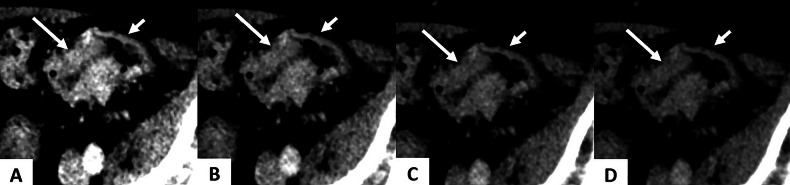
54-year-old male with pT3 sigmoid adenocarcinoma. DS-DECT (Siemens Force in axial section at VP with VMI at different energy levels. A 40 keV, B 50 keV, C 60 keV, D 70 keV. 40 keV VMI image shows improved contrast between high enhancement of the tumor (long arrow) and adjacent enhancement of normal bowel (short arrow). DS-DECT, dual-source dual-energy computed tomography; ROI, region of interest; VMI, virtual monoenergetic images; VP, venous phase
